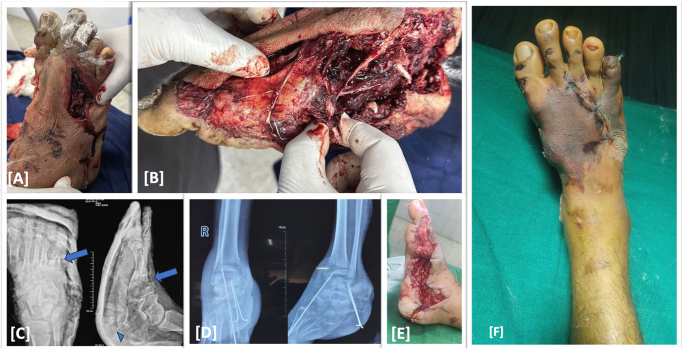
Crush injury of Right foot with lacerated skin and exposed muscle and bony fragments (A and B); Plain radiograph showing comminuted medial, middle, and lateral cuneiform fractures, 4th and 5th Meta-tarsal fractures (blue arrows), and calcaneus chip fracture(arrowhead) (C); post-operative radiograph showing K-wire stabilization (D); Post-debridement status with skin tag suturing (E); Clinical image showing evidence of infection with blackening of skin, more pronounced at 5th toe (F).
